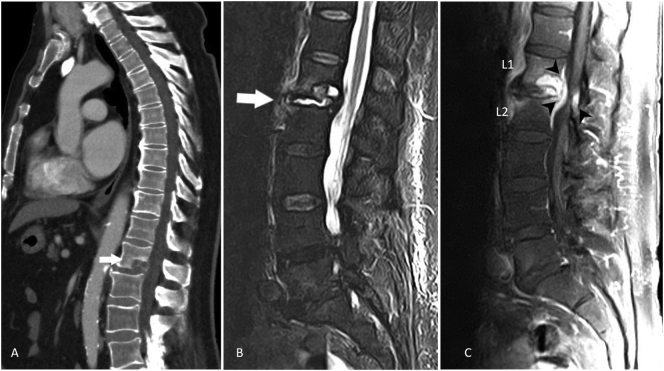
A: A sagittal computed tomography (CT) scan of the thoracolumbar spine shows a suspect lytic lesion in the dorsal region of the first lumbar vertebra (white arrow pointing towards L1). B: preoperative sagittal STIR T2 weighted magnetic resonance imaging (MRI) showing a hyperintense fluid signal within the disc at L1 and L2 (white arrow). C: MRI (T1, sagittal, post contrast medium) shows a significant contrast enhancement of the affected area, with a posterior extension, narrowing the spinal canal (black arrowheads).
